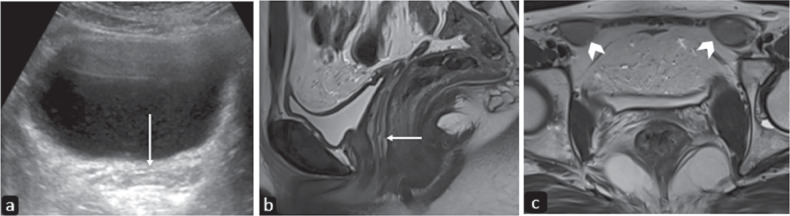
Imaging features of androgen insensitivity syndrome. USG image in the transverse plane (a) of a seventeen-year-old phenotypic female with primary amenorrhea and 45XY karyotype reveals a linear echogenic structure posterior to the bladder likely vaginal canal with no evidence of any uterine tissue or ovaries. T2 weighted sagittal MR image (b) demonstrates a linear hyperintense vaginal canal (arrow) between the urinary bladder anteriorly, rectum, and anus posteriorly. Note the absence of a cervix or uterus. T2W axial image at the level of the inguinal canal (c) shows bilateral testes with epididymis seen in the inguinal region (arrowheads)
